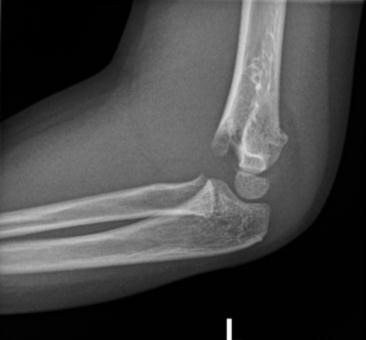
Lateral radiograph of Wilkin’s-modified Gartland Grade 2b supracondylar humeral fracture.
